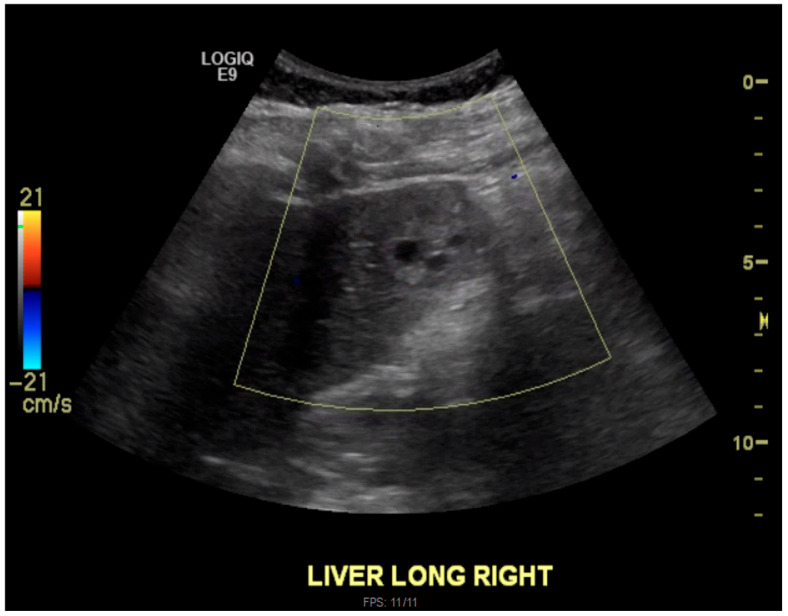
Liver ultrasound. Longitudinal section. Right lobe.
